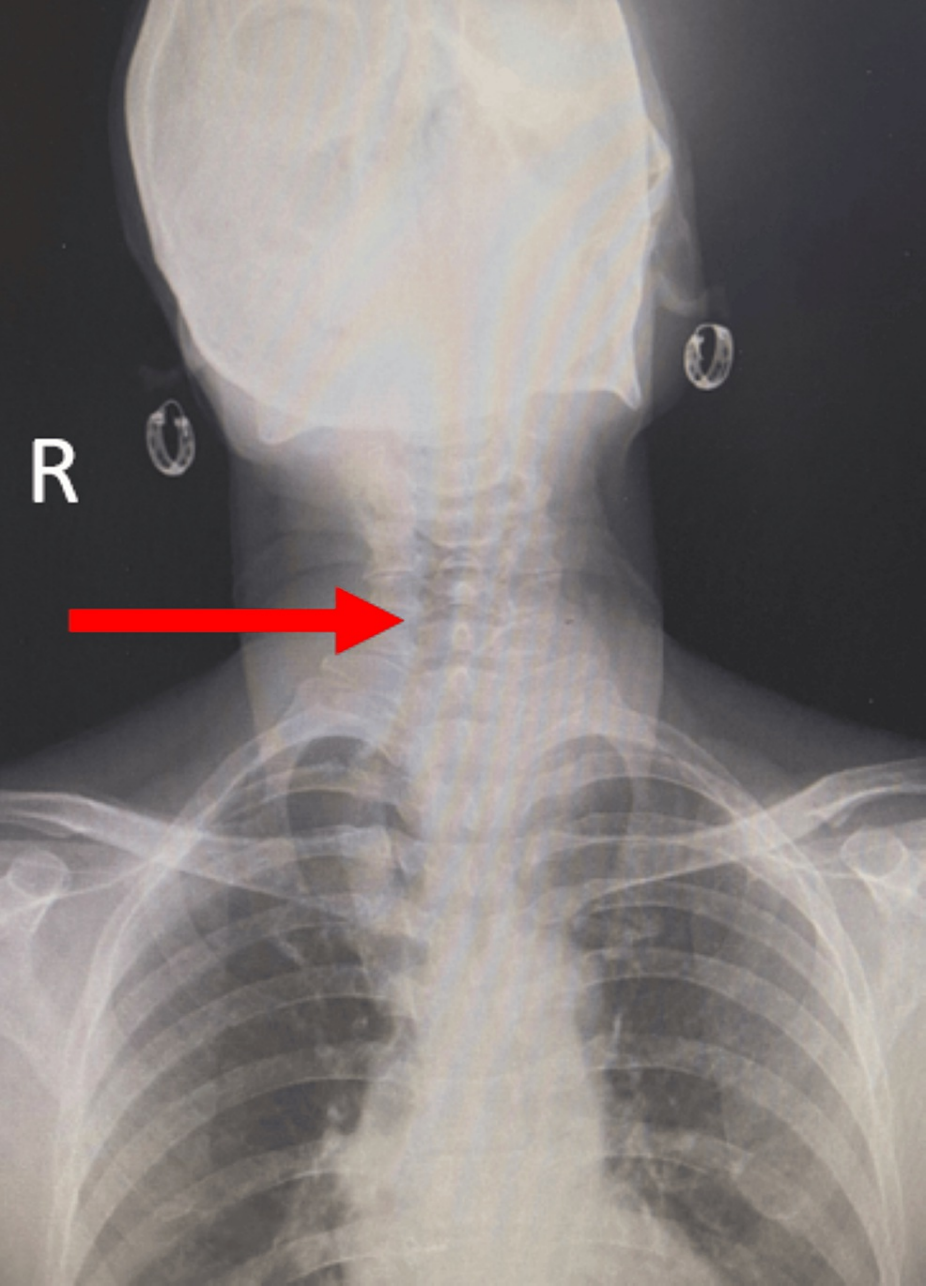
X-ray showing tracheal deviation to the right (as shown in red arrow)
